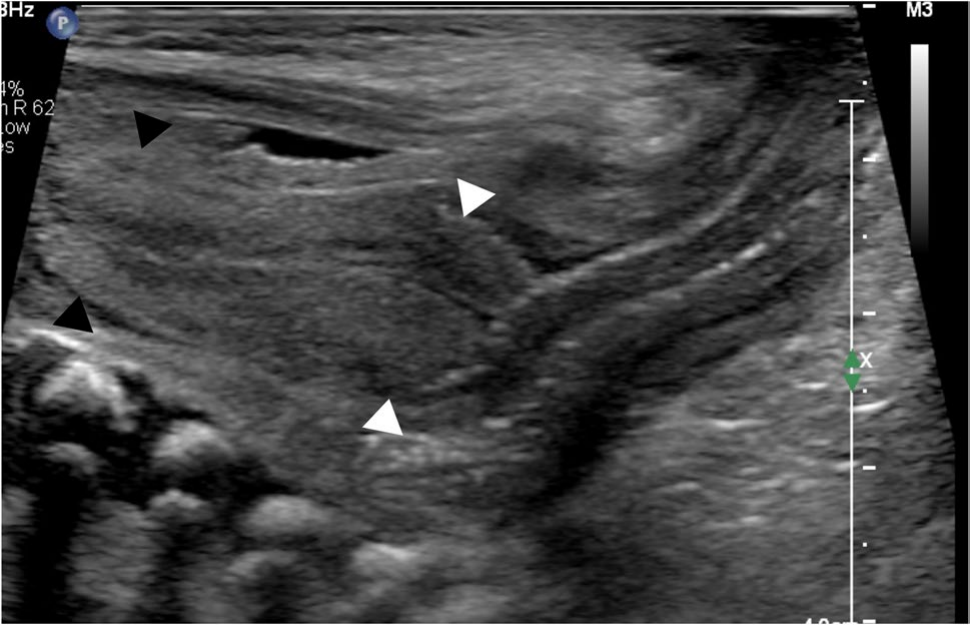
Sagittal gray-scale ultrasound image from a perineal approach in a female neonate shows a spade-shaped uterus, the cervix (*white arrowheads*) being wider than the fundus (*black arrowheads*)
